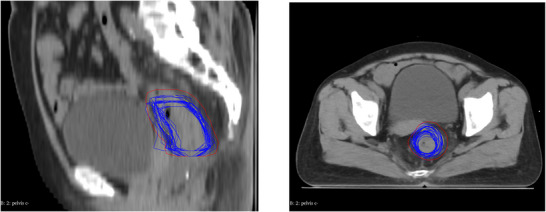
GTV displacement of patient 8. Both the sagittal (left) and axial (right) views are taken at the level of the maximum tumor volume. The planning GTV (yellow) is delineated on the planning CT, and PGTV (red) is generated with a 6 mm expansion. All the GTVs (blue) contour from the FBCT were mapped to the planning CT after rigid registration with respect to bony anatomy.
